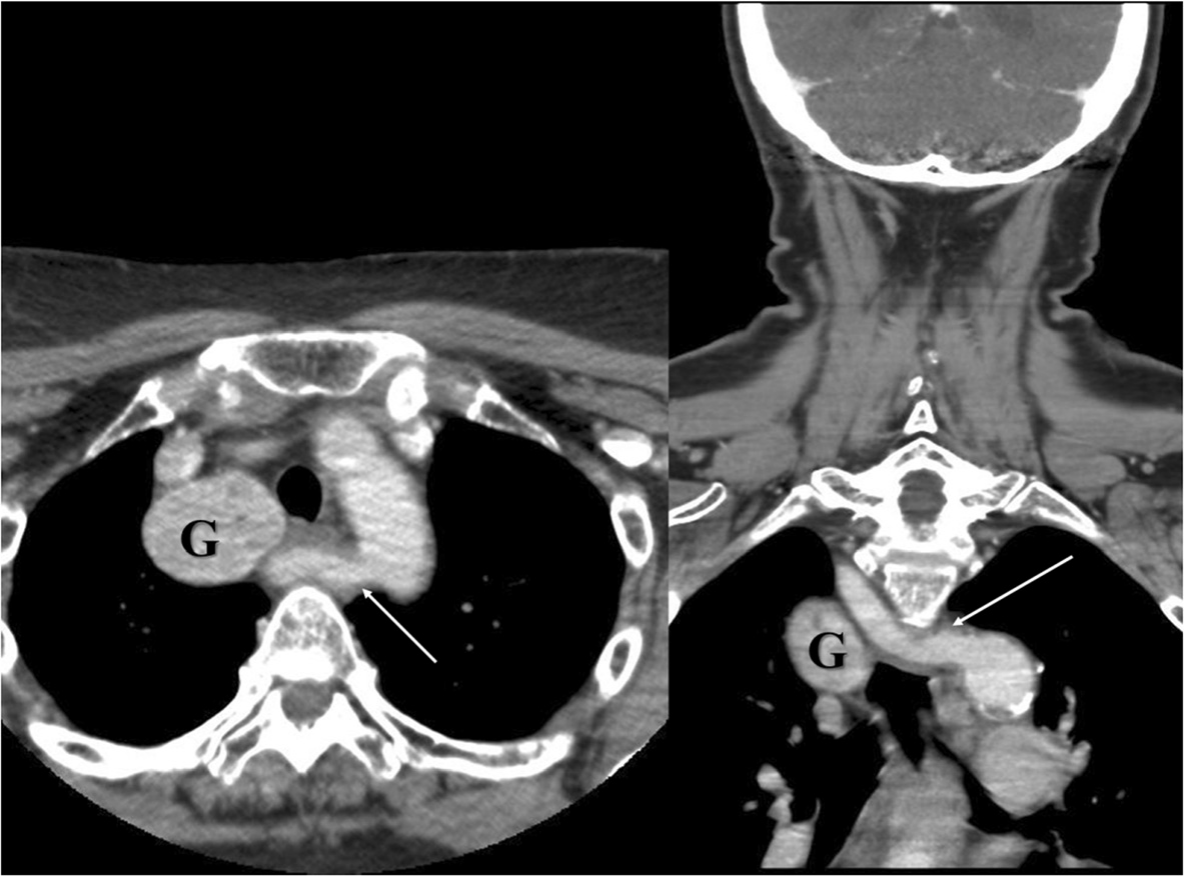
Preoperative CT scan indicating a mediastinal goitre (G) and an aberrant right subclavian artery (white arrow).
